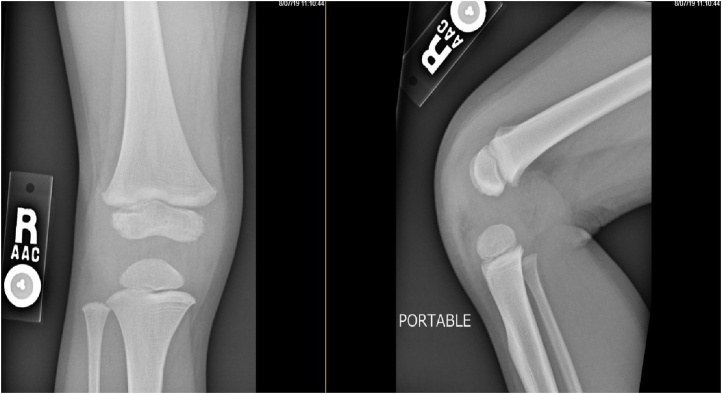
Radiographs of the right knee taken upon the patient's initial evaluation to the emergency department. No osseous or soft tissue abnormalities are noted.
